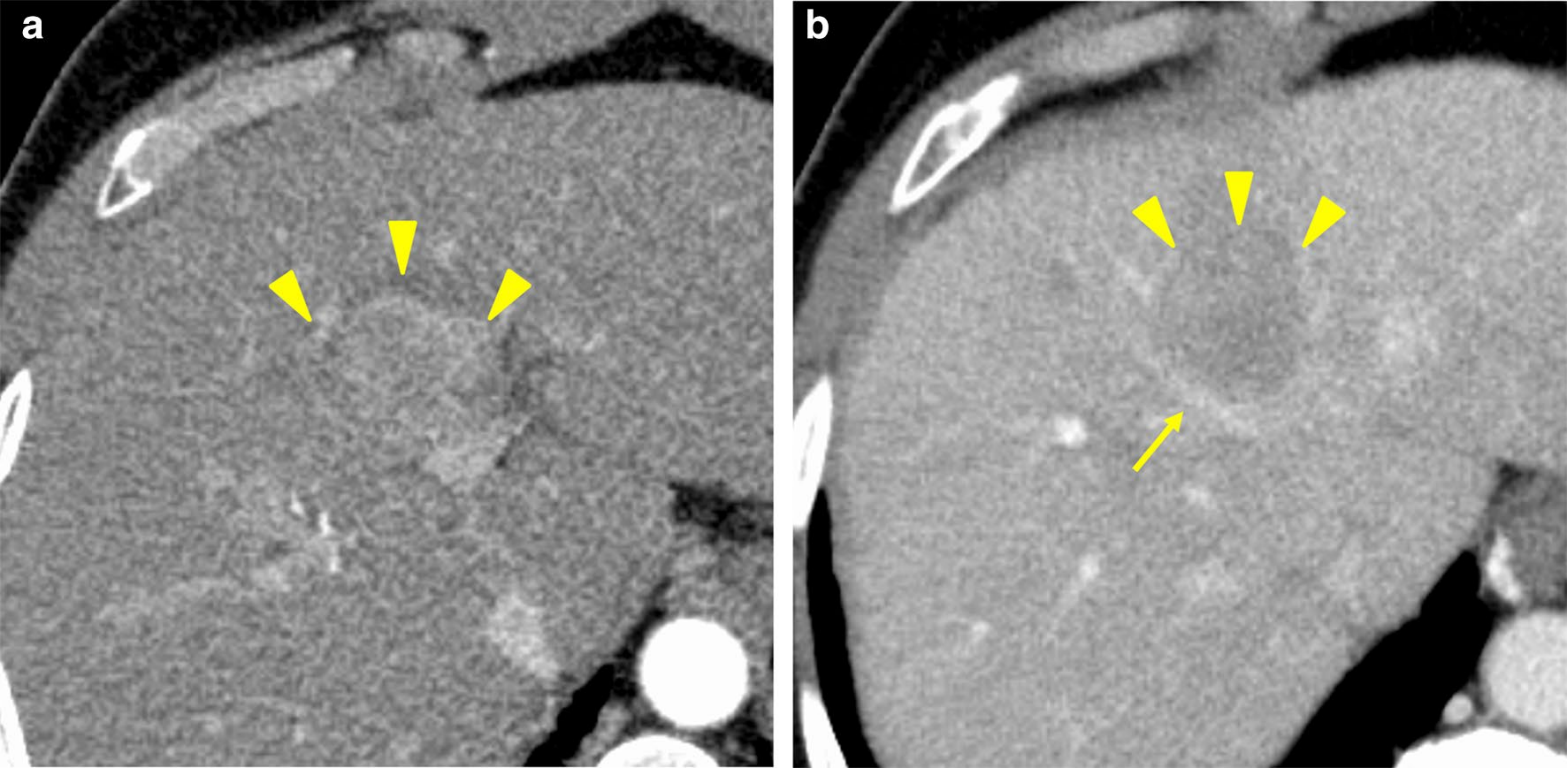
Preoperative contrast-enhanced computed tomography image of the liver. The tumor was located in the medial section of the liver (arrowheads) and showed heterogeneous enhancement in the arterial phase (a) and washout in the portal venous phase (b). The arrow indicates the middle hepatic vein
